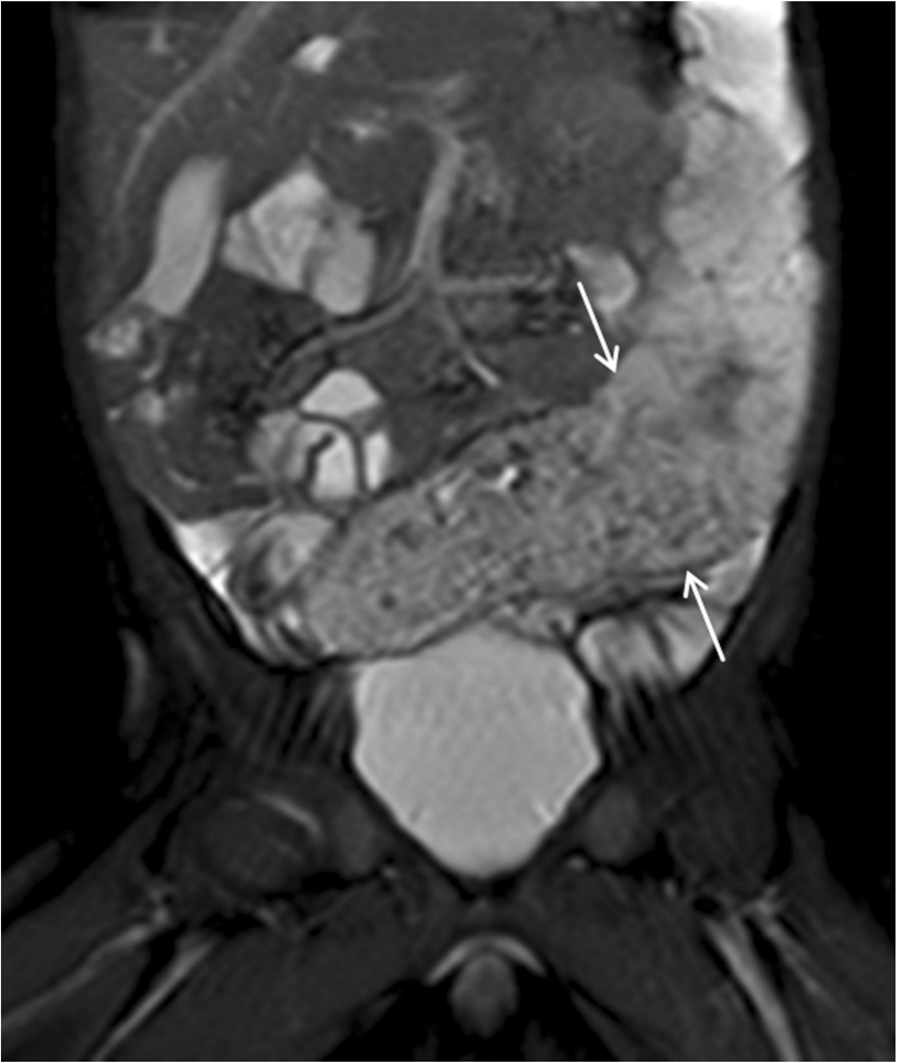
MRI: coronal TruFISP image demonstrating the vascular malformation (arrows).
